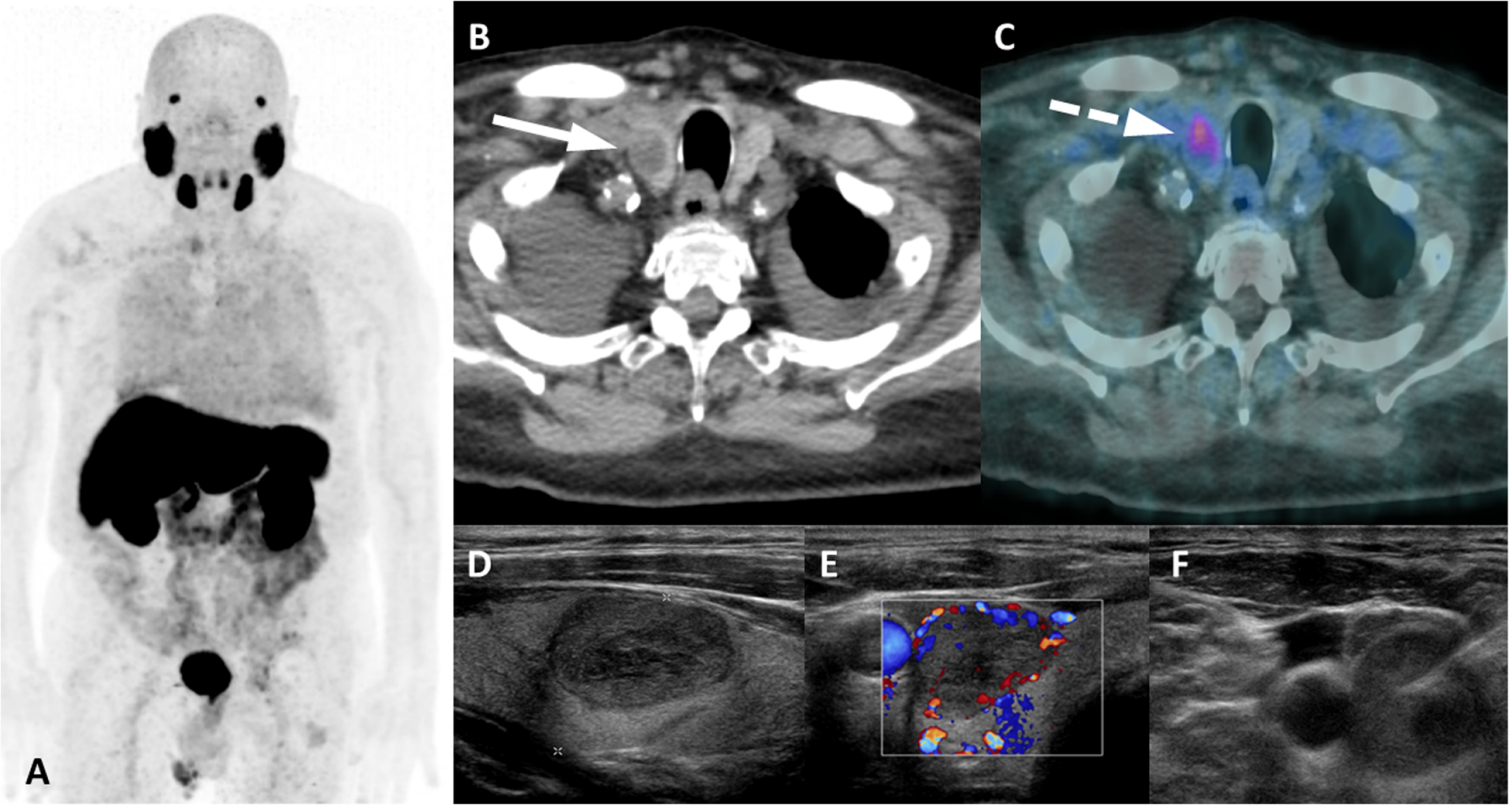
Thyroid benign adenoma with PSMA uptake. ^68^Ga-PSMA-PET MIP (a), axial CT (b) and axial PET/CT (c) images show nodulae with mild uptake (dashed arrow) in the right thyroid lobe (arrow). Thyroid ultrasound revealed a hypoecogenic nodule with cystic areas (d) and peripheric vascularization (d). Fine needle aspiration of the thyroid nodule (e) revealed follicular adenoma without malignant cells
